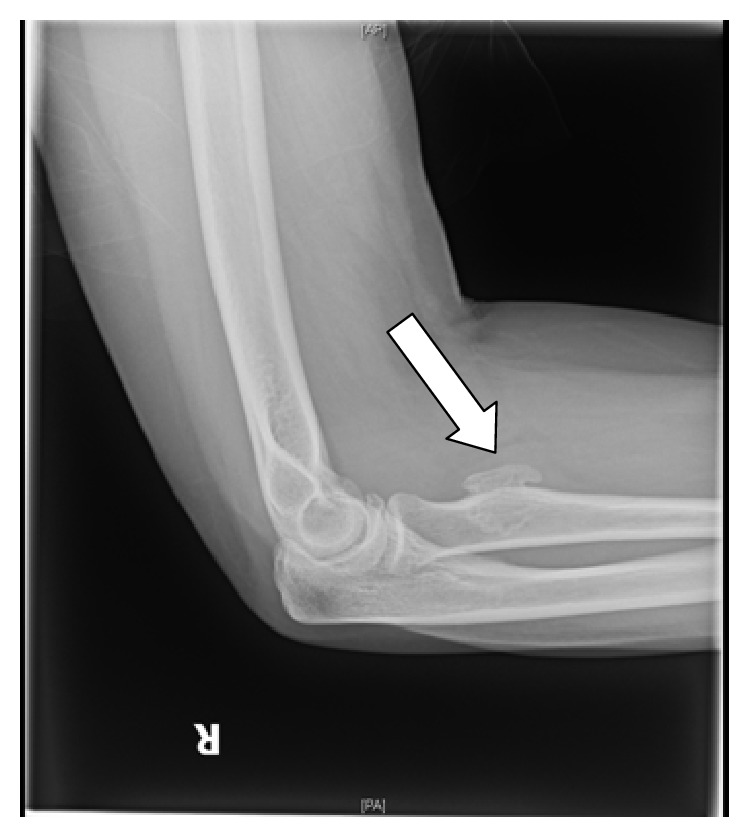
Plain radiograph reported this calcified area as an osteochondroma right forearm (fat arrow).
